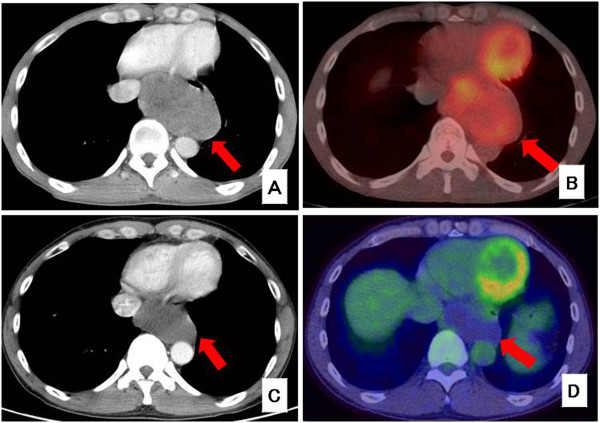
CT and PET-CT images. (A) CT scan before chemotherapy. The tumor was 92 × 55 mm × 80 mm in diameter. (B) PET-CT scan before chemotherapy. SUV max was 4.9. (C) CT scan after chemotherapy. The tumor was 70 mm × 37 mm × 60 mm in diameter. (D) PET-CT scan after chemotherapy. SUV max was 1.2.
